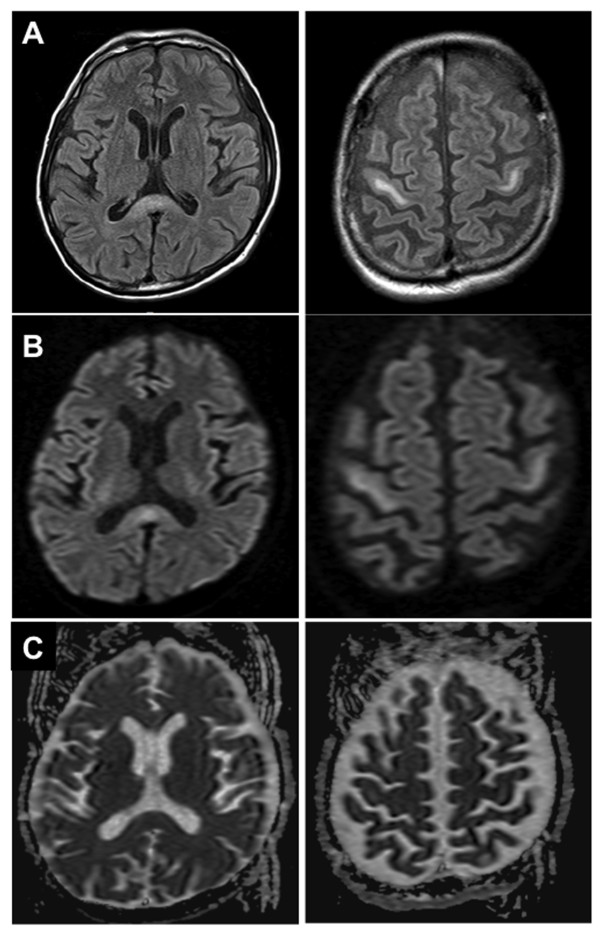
Admission MRI. Representative images of brain MRI on admission including fluid attenuated inversion recovery (FLAIR) (A), diffusion-weighted image (DWI) (B), and the apparent diffusion coefficient (ADC) map (C), showing hyperintense lesions in the precentral gyrus bilaterally and the splenium of the corpus callosum.
